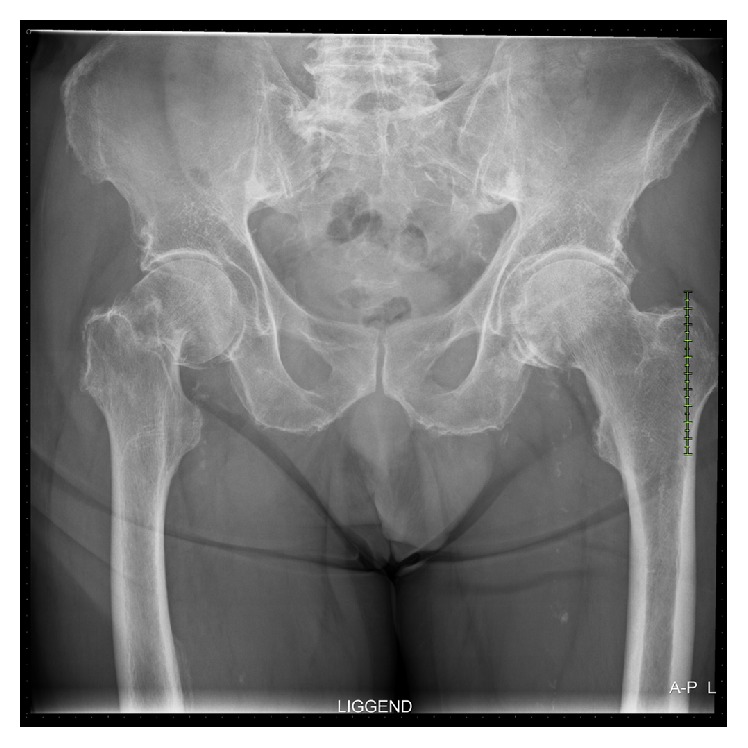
Anterior-posterior X-ray pelvis: displaced neck of femur fracture (right).
